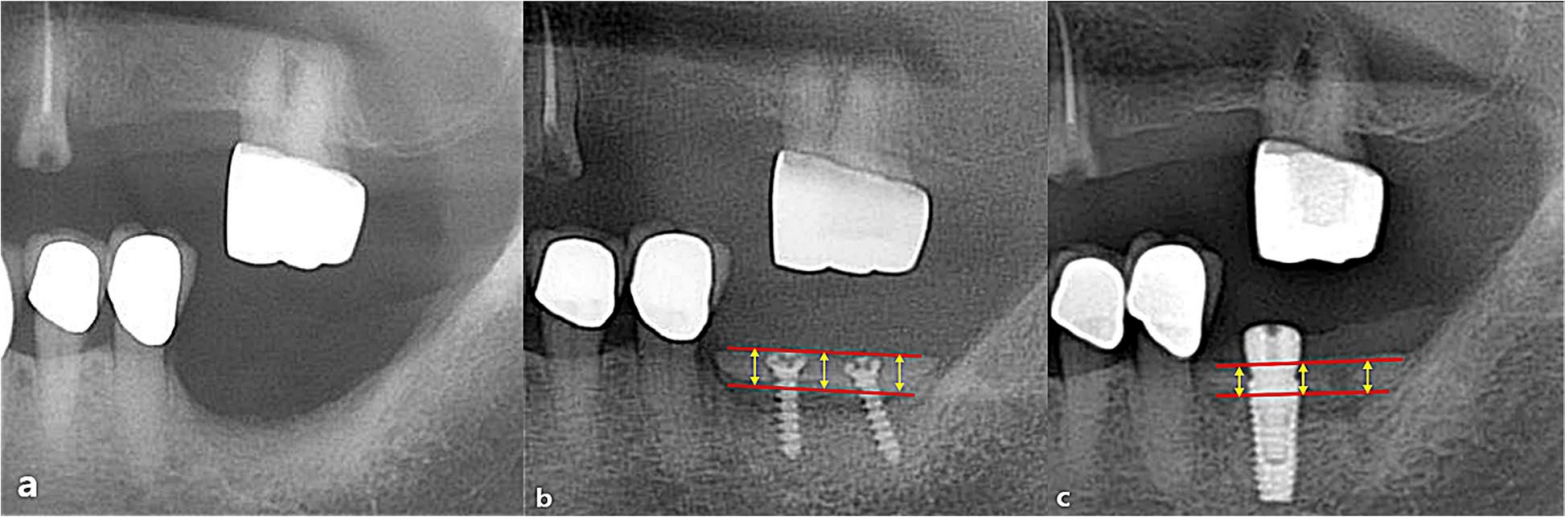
Measurement of the vertical amount of bone gain and resorption amount. a Vertical alveolar bone gain was measured using an immediate post-operative radiograph, calculating the distance between the adjacent tooth and the top of the grafted bone or the head of the screw. b Three months after augmentation, the extent of vertical bone resorption was assessed
